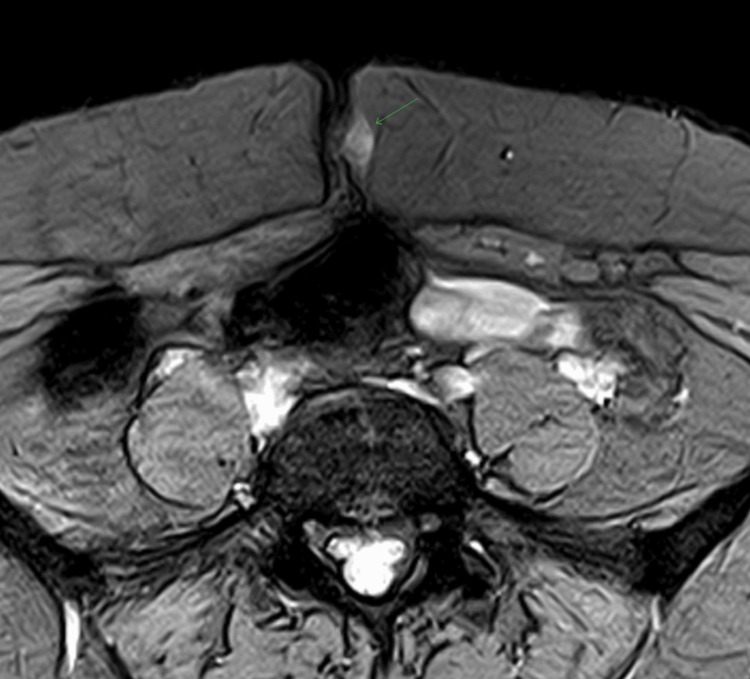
Axial T2 FS MRI image showing a hyperintense signal intensity subcutaneous lesion (arrow) within the umbilicus. No intraperitoneal extension is seen.
